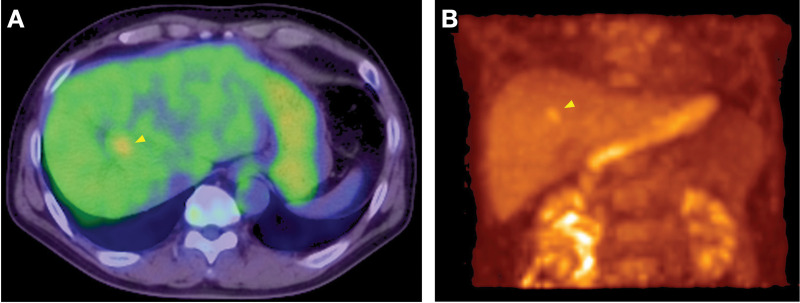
Fluorodeoxyglucose (FDG)-PET/CT findings. (A) FDG-PET/CT showing abnormal uptake along the intrahepatic bile duct from segment 8 to the hepatic hilum (SUVmax 3.6–4.1). (B) Maximum intensity projection image confirming absence of lymph node or distant metastasis. Arrowheads indicate the location of the intraductal lesion in all panels.
